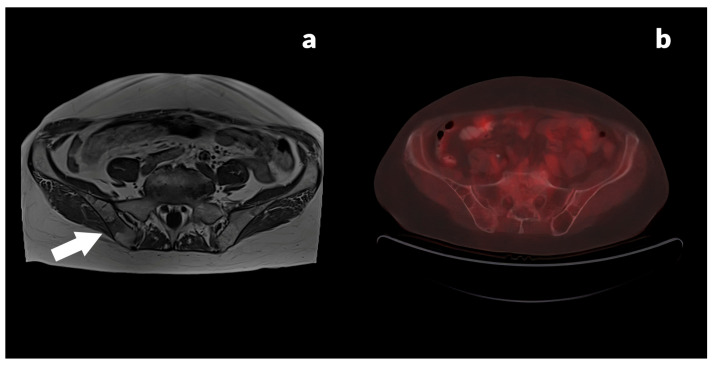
(a) Axial T1W MR image shows focal T1W hypointense lesion in the right iliac bone (white arrow) corresponding with metastasis, and (b) PET/CT axial image does not detect FDG-avid lesion in the region of pelvic bone.
